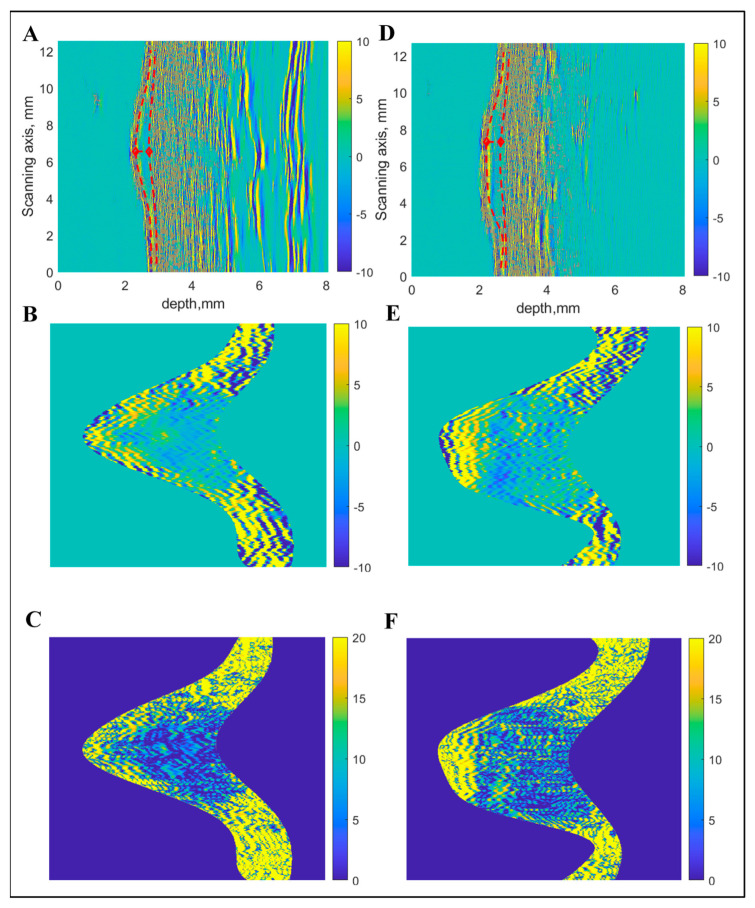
Boundary detection and extraction of region of interest from ultrasonic B-scan: Raw B-scan image (A), extracted region of interest with linear scale of amplitudes (B) and with logarithmic scale (C) in the case of melanocytic naevus (MN). Raw B-scan image (D), extracted region of interest with linear scale of amplitudes (E) and with logarithmic scale (F) in the case of cutaneous melanoma (CM).
